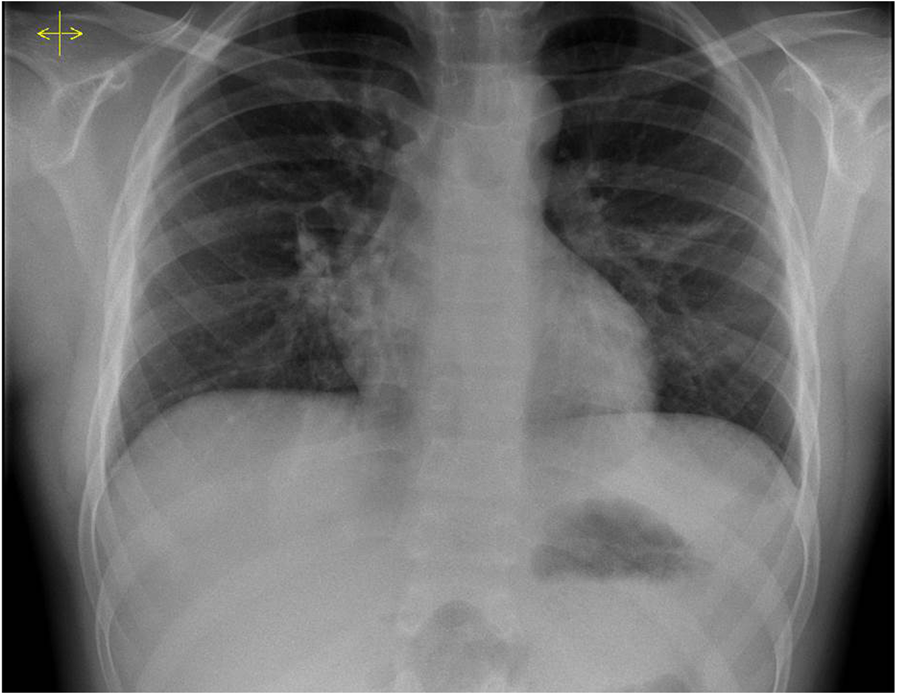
Chest x-ray at follow-up 2 months after surgical repair of the diaphragmatic defect. Note normal position of the stomach bubble and the normal diaphragmatic contour
